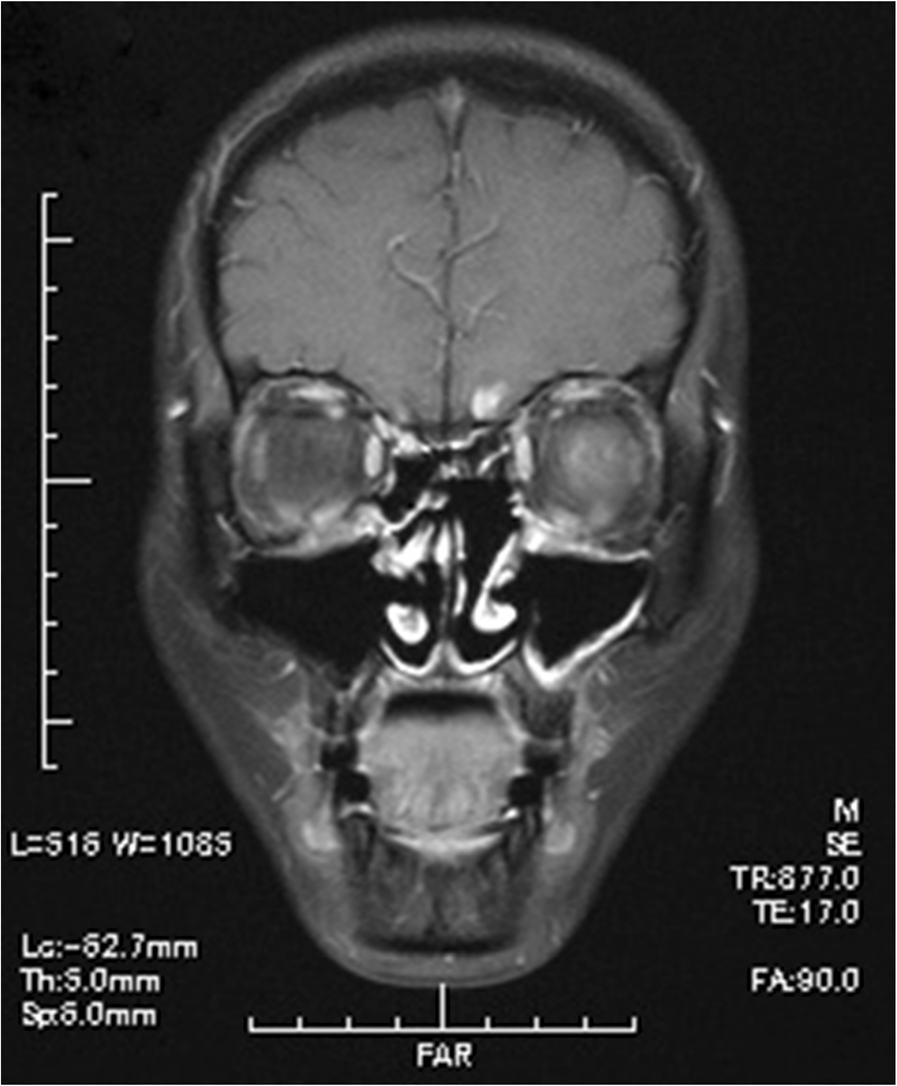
Coronal view of follow-up MRI 10 years after radiotherapy (patient 1). Focal enhancement at the base of the frontal lobe was observed. This finding disappeared in follow-up MRI after 9 months.
